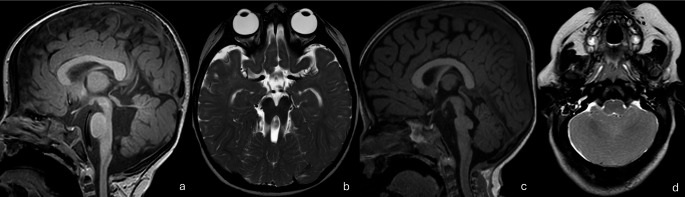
(a, b) Joubert syndrome. Thickened, horizontally oriented superior cerebellar peduncles and vermian hypoplasia (A), along with a deep interpeduncular fossa producing the classic “molar tooth sign” on axial MRI (B) (c) Tegmental cap dysplasia. Dorsal “cap-like” bulge of the pontine tegmentum, and dysplastic brainstem, and vermo-cerebellar hypoplasia/dysplasia (d) Rhombencephalosynapsis*.* Agenesis of the cerebellar vermis with fusion of the cerebellar hemispheres and dentate nuclei, resulting in a characteristic midline “fused cerebellum” appearance
